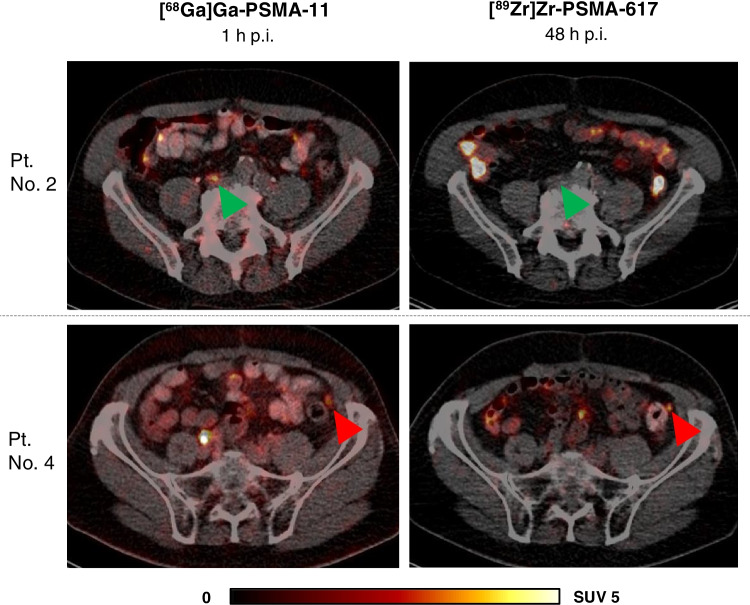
Transversal slices of [^89^Zr]Zr-PSMA-617 (right) and [^68^Ga]Ga-PSMA-11 (left) PET/CT of 2 patients (patient no. 2 and 4) with undetermined PSMA-positive findings on [^68^Ga]Ga-PSMA-11 PET/CT clarified by [^89^Zr]Zr-PSMA-617 PET/CT. Green arrows point to pelvic tracer uptake (SUV_max_ 4.47) on [^68^Ga]Ga-PSMA-11 PET/CT 1 h p.i. with no corresponding uptake on [^89^Zr]Zr-PSMA-617 PET/CT, considered as unspecific uptake. Red arrows point to faint tracer uptake (SUV_max_ 2.06) in a peritoneal lesion on [^68^Ga]Ga-PSMA-11 PET/CT 1 h p.i. with corresponding uptake (SUV_max_ 2.87) on [^89^Zr]Zr-PSMA-617 PET/CT 48 h p.i. considered as peritoneal metastasis
